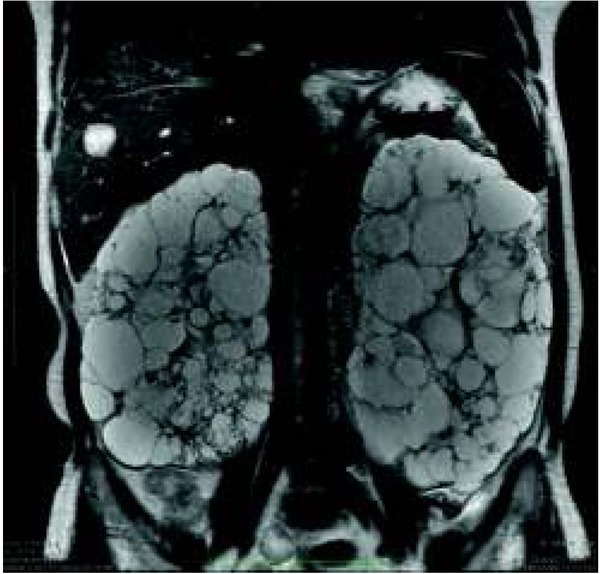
Magnetic resonance imaging, T2-weighted images. Polycystic kidney disease. Cyst in the liver. Ryc. 9. Obrazowanie metodą rezonansu magnetycznego, obrazy T2 zależne. Wielotorbielowatość nerek. Zmiana torbielowata wątroby.
